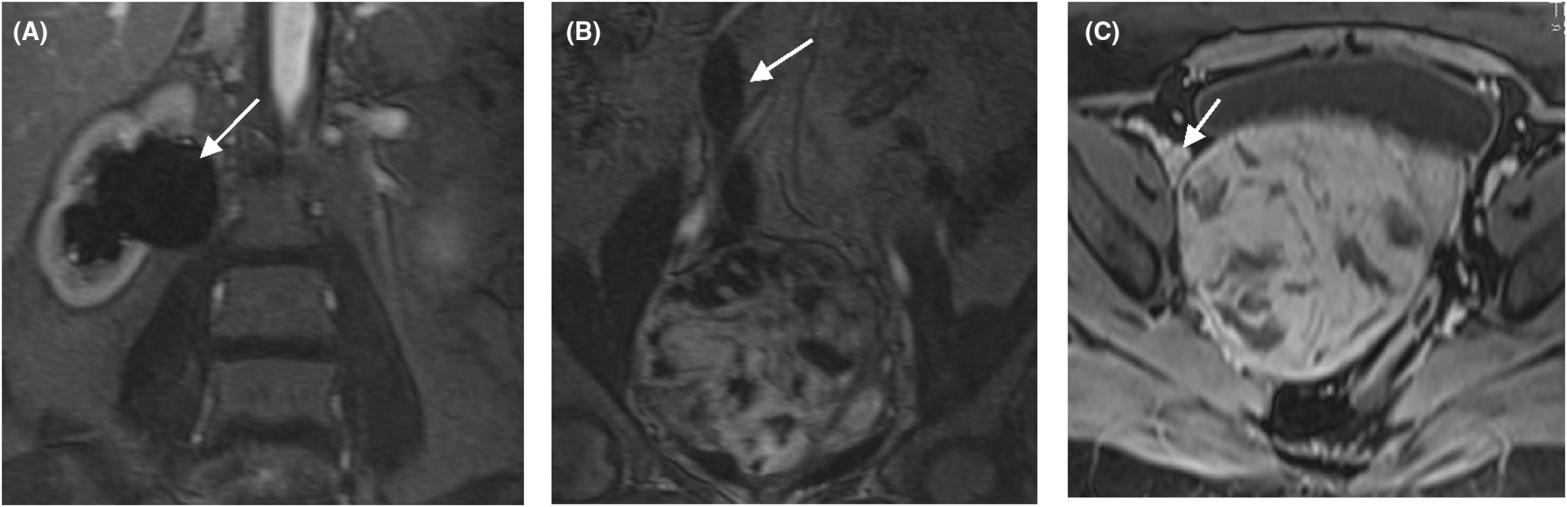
Coronal and axial gadolinium‐enhanced T1‐weighted fat‐suppressed (A–C) MR images show right side hydroureteronephrosis (arrow on A and B) and right external iliac vein (arrow on C).
